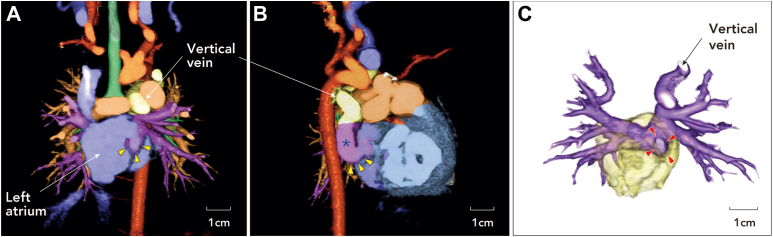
Preoperative 3-dimensional volume rendering of computed tomography images taken at the age of 5 months, before the appearance of the pulmonary venous obstruction. (A) Coronal image and (B) sagittal image showing the tubular connection (arrowheads) between the pulmonary venous confluence (asterisk) and the left atrium. (C) The entire image of the pulmonary venous system viewed from the front, with the posterior wall of the left atrium drawn in transparent yellow. The orifice of the connection is indicated by arrowheads.
